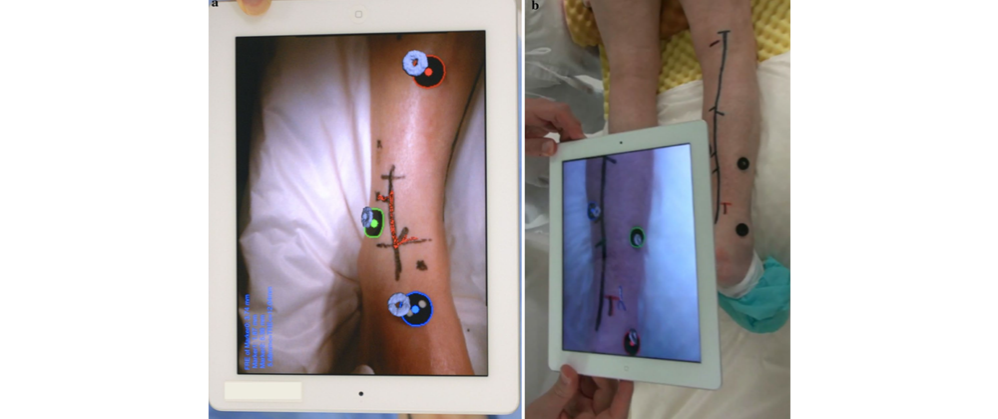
Computed tomography–derived 3D model is superimposed onto camera images. Doughnut-shaped fiducial markers on the limb help match images so that the target artery and its branches can be overlaid onto the limb as red (A) or blue (B) lines. Reprinted by permission from the Springer Nature Customer Service Centre GmbH: Springer Nature. New simple image overlay system using a tablet PC for pinpoint identification of the appropriate site for anastomosis in peripheral arterial reconstruction. Mochizuki Y, Hosaka A, Kamiuchi H, Nie JX, Masamune K, Hoshina K, et al. 2016 [40].
